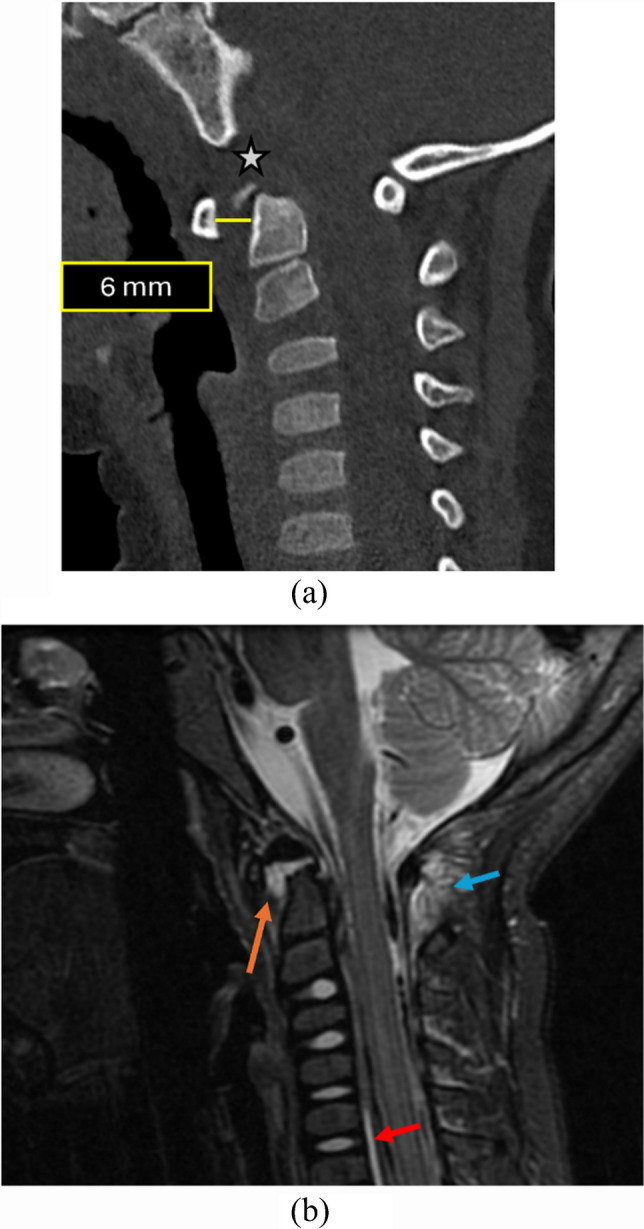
A 3-year-old child in a motor vehicle accident. (a) Sagittal CT of the cervical spine revealed fracture of dens (*star*) with widened anterior atlanto-dental interval measuring 6 mm (normal <2.6 mm). (b) MRI cervical spine performed soon after showing fluid in the anterior atlanto-dental interval (*orange arrow*). Also, ligamentous injury evidenced by edema is seen in the interspinous spaces at C1-2 (*blue arrow*). There is fluid also seen extending along the posterior longitudinal ligament (*red arrow*)
